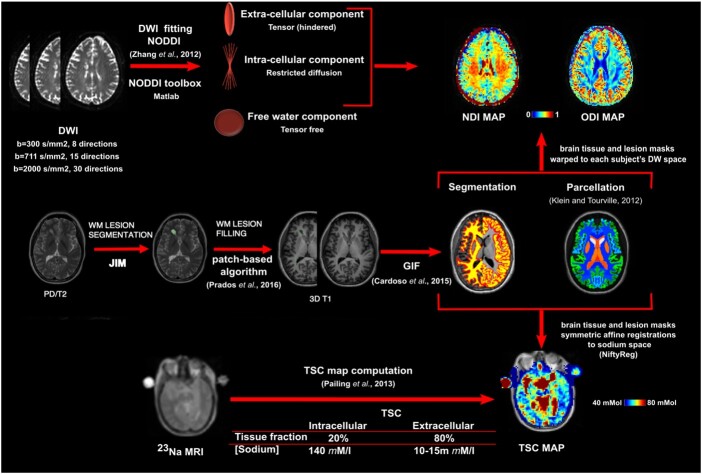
MRI post-processing. MRI post-processing for lesion masks, NODDI and ^23^Na MRI (images from a patient presented in the native space for each modality). DWI = diffusion-weighted imaging; GIF = geodesic information flow; PD = proton density; WM = white matter.
